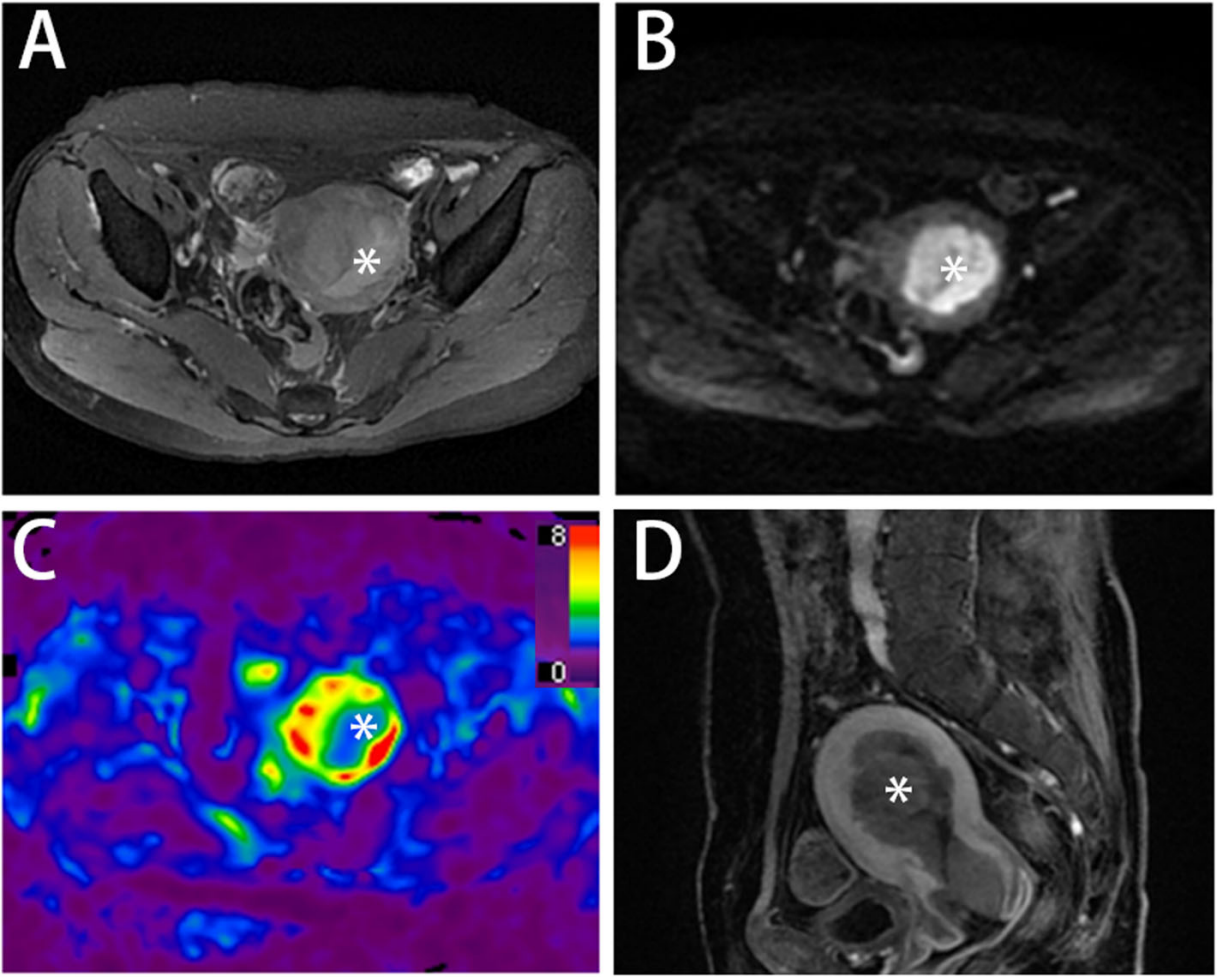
A 55-year-old woman presented with abnormal vaginal bleeding and underwent pelvic MRI and MRE scans. Axial T2-weighted image (A), diffusion-weighted image (B), and sagittal T1-weighted image with contrast (D) showed a mass (4.5×3.6 cm, asterisk) in the uterus. Tumor stiffness was 3.08 kPa measured by 3D MRE elastograms (C) at 60 Hz. Serous papillary adenocarcinoma (Type II) with deep myometrial invasion was surgically confirmed
